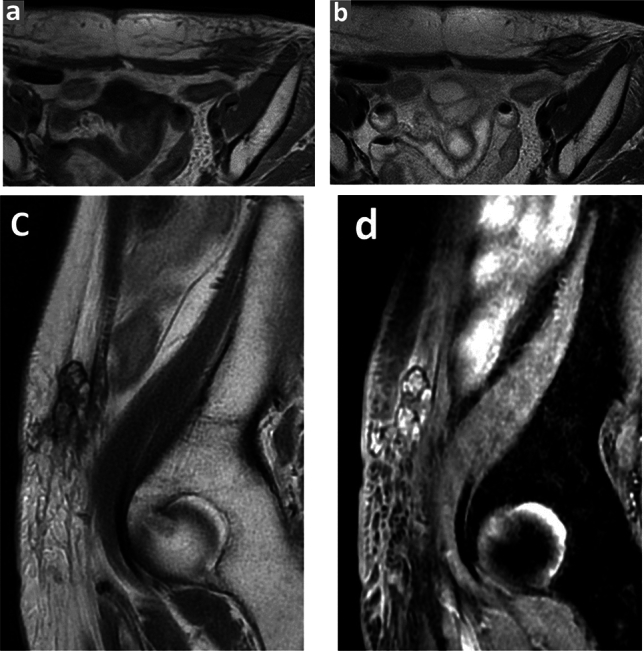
A 77-year-old female with a 10-year history of lymphedema following radical hysterectomy with pelvic lymphadenectomy for cervical cancer, presented with a palpable mass of the left lower abdominal wall (case 5). a and b Axial T1 and T2-weighted images show an ill-defined subcutaneous mass in the lower abdominal wall. c and d Sagittal T2- and fat-suppressed T1-weighted images show a confluent multinodular shaped mass with a mixture of intermediate and high signal intensities
